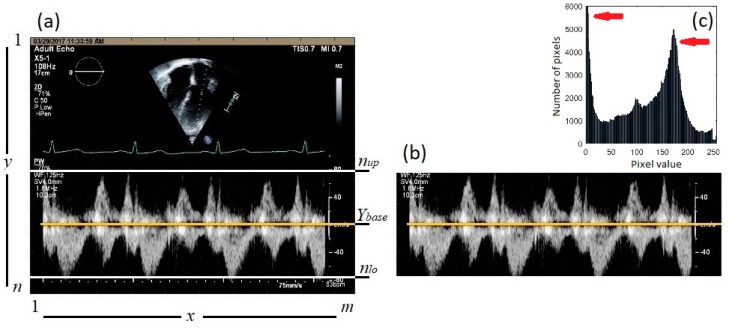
(a) A sample Doppler echocardiography image. The horizontal and vertical axes represent the number of pixels. The upper and lower borders of the region of interest (ROI) are marked with white solid lines. The baseline is shown with a yellow solid line. (b) The resulting image after removing the image portions outside the ROI. (c) Histogram of the ROI. The red arrows show the main peaks of the histogram which were used to determine the threshold values.
